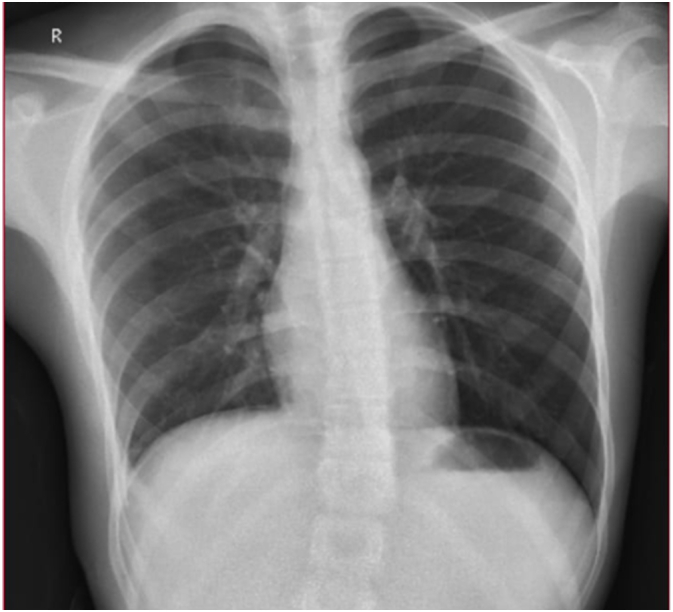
Post-operative day one, chest X-ray showed no abnormalities.
